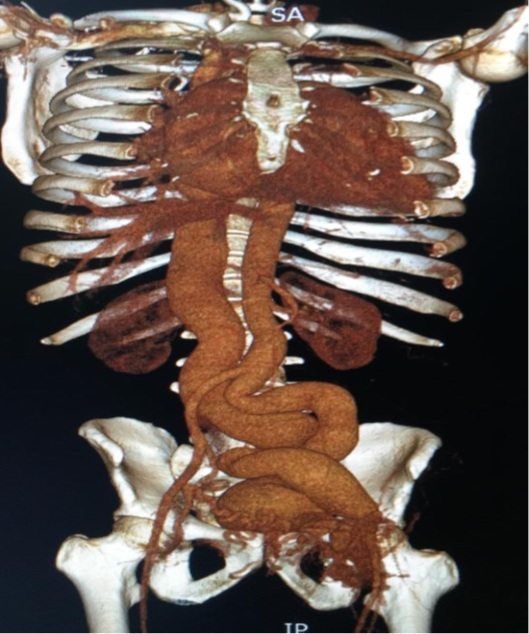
Three-dimensional reconstruction from computed tomography angiography showing dilation of the iliac vessels, inferior vena cava, intrapelvic veins, and veins of the left inguinal region.
